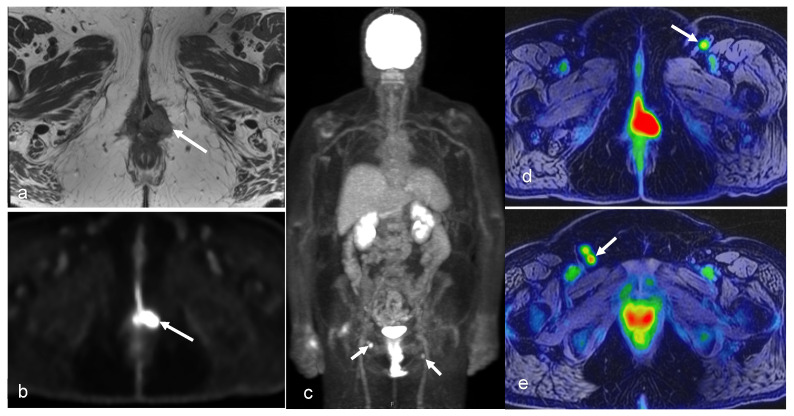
A 64-year-old woman with history of vulvar cancer diagnosed 4 years prior and initially treated with left vulvectomy. The patient was re-evaluated due to new symptoms of pain and itching, and a biopsy was performed, revealing a recurrent high-grade squamous neoplasm. PET/MRI was ordered for restaging. Axial PET (b) and T2-weighted (a) images from dedicated pelvis MRI with dedicated pelvic PET show an FDG-avid, nodular, intermediate T2 signal in the left perineum compatible with local recurrence. The whole-body survey MIP image (c) and axial-fused PET/MRI images (d,e) demonstrate bilateral FDG-avid inguinal lymph nodes without more distant metastatic disease. The patient went on to undergoing wide local excision with adjuvant radiation and chemotherapy. (Courtesy of Eric C. Ehman, MD, Department of Radiology, Mayo Clinic, Rochester, MN, USA).
